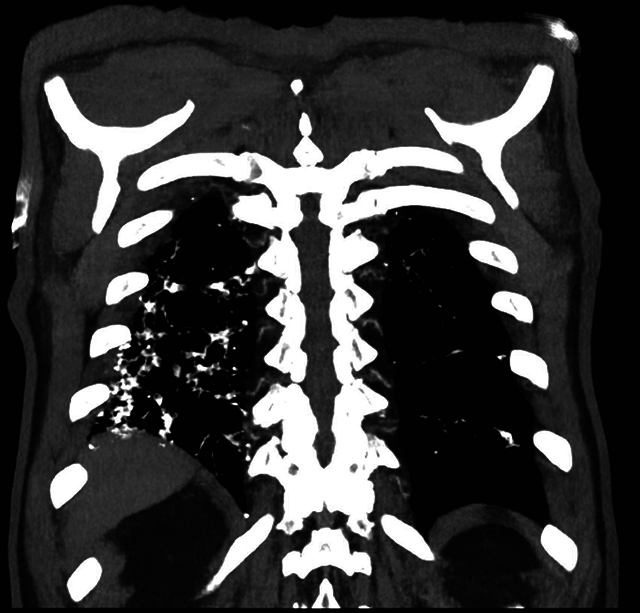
Most commonly, patients with DPO are asymptomatic and are often diagnosed in a postmortem examination. Therefore, on CT, DPO is almost always an incidental finding. They might exhibit a mild cough or dyspnea; however, in most cases, this is due to the pre-existing lung condition (such as chronic obstructive pulmonary disease [COPD] or ILDs such as UIP or nonspecific interstitial pneumonia [NSIP]).
